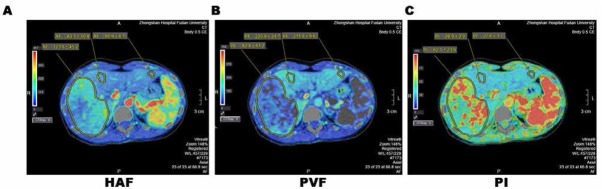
A 37-year-women was diagnosed as advanced HCC. A to C, Liver perfusion CT maps of HAF, PVF, and PI, respectively. The backrground liver parenchyma HAF was 83.3±10.8 and 80.9±6.1mL per 100 mL/min, HCC PVF was 123.0±45.2 mL per 100 mL/min.
